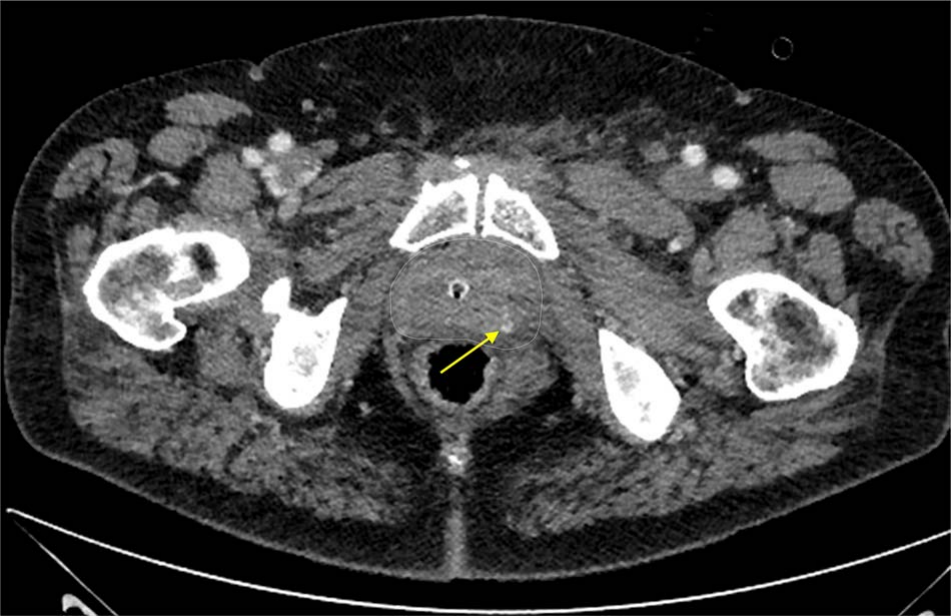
Contrast-enhanced CT scan of the prostate showing focal extravasation in the left dorsolateral midgland, suggesting slow venous oozing. The prostate contour is marked by a dashed line.
